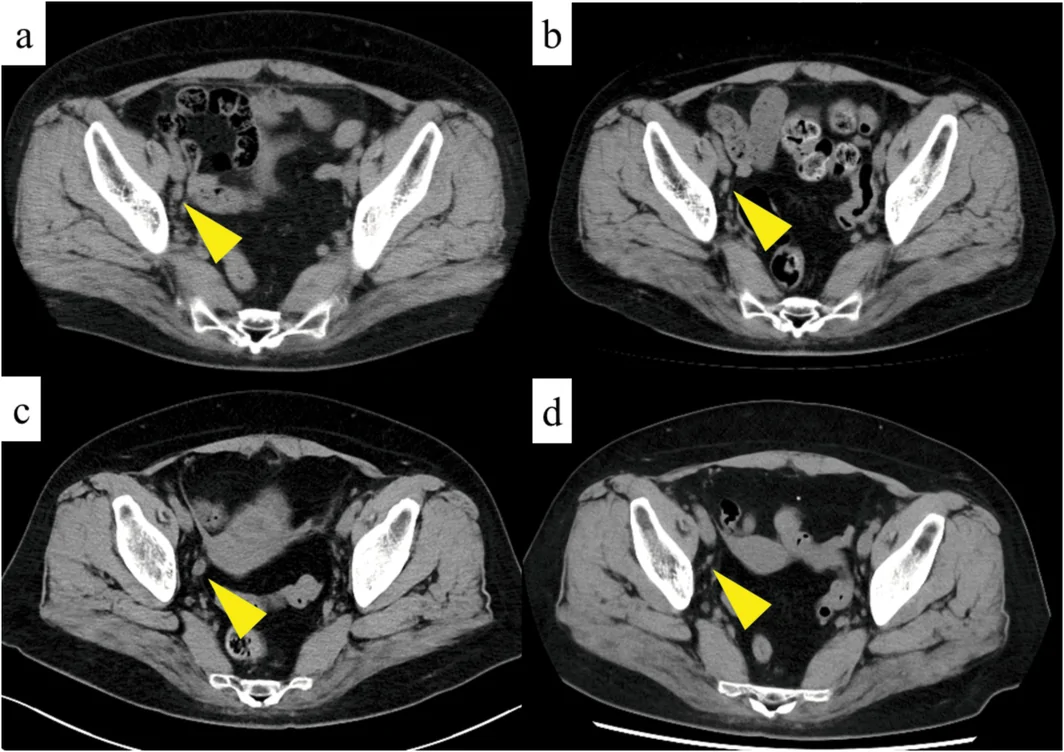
Sequential radiologic changes of the right obturator lymph node metastasis. (a) Baseline axial CT showing an enlarged right obturator lymph node. (b) After four cycles of gemcitabine–cisplatin, the right obturator lymph node showed slight interval reduction; however, the overall radiologic response remained stable disease. (c) After seven cycles of gemcitabine–cisplatin, the lesion enlarged compared with the post‐treatment nadir, fulfilling radiologic criteria for progressive disease. (d) Following pembrolizumab initiation, the right obturator lymph node decreased from 20 mm in short‐axis diameter at treatment initiation to 5 mm at 12 months, corresponding to a 75% reduction and a partial response according to RECIST 1.1.
